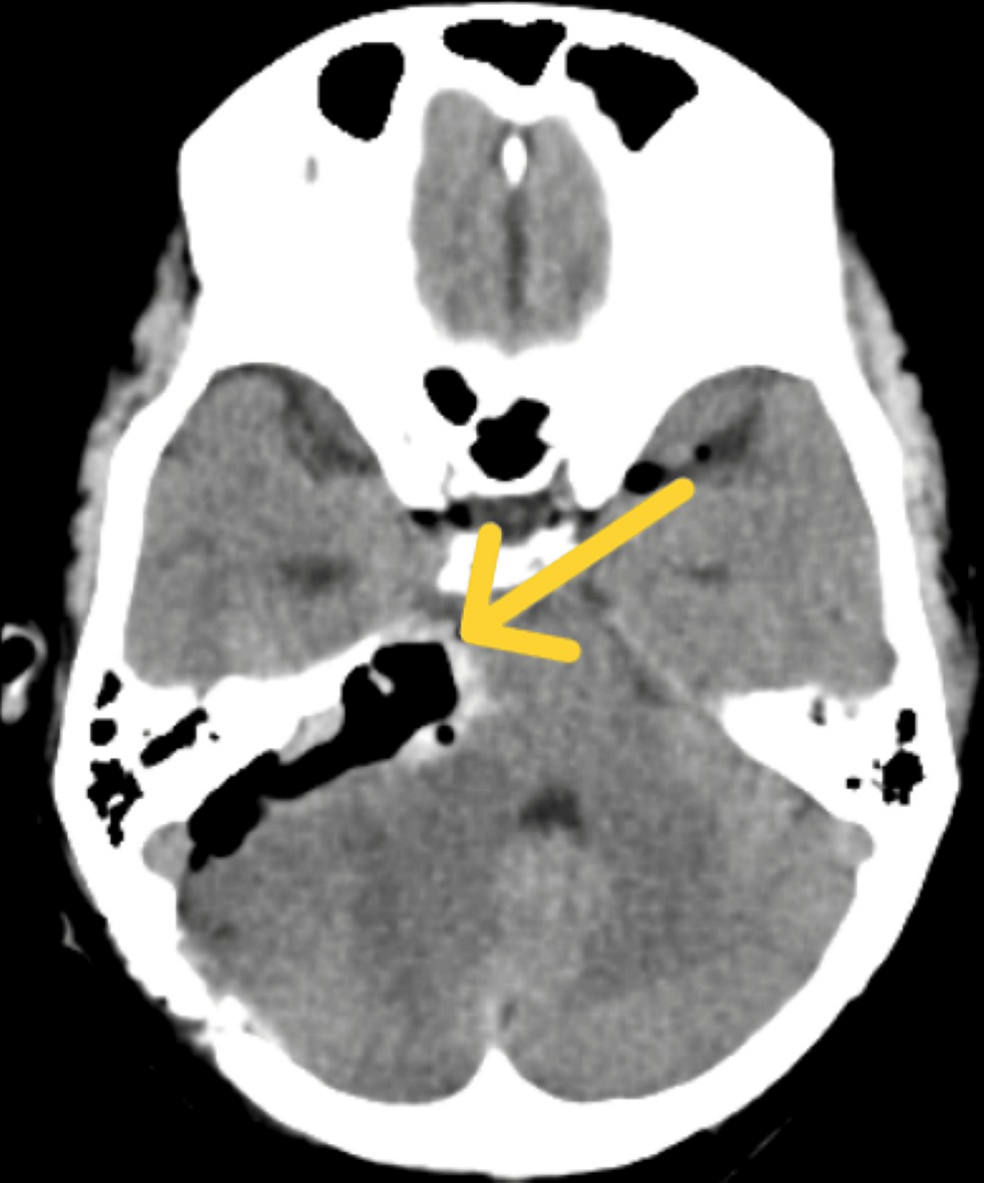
CT scan of the patient showing tumour resection The yellow arrow shows the site of tumour resection. CT: computed tomography.
